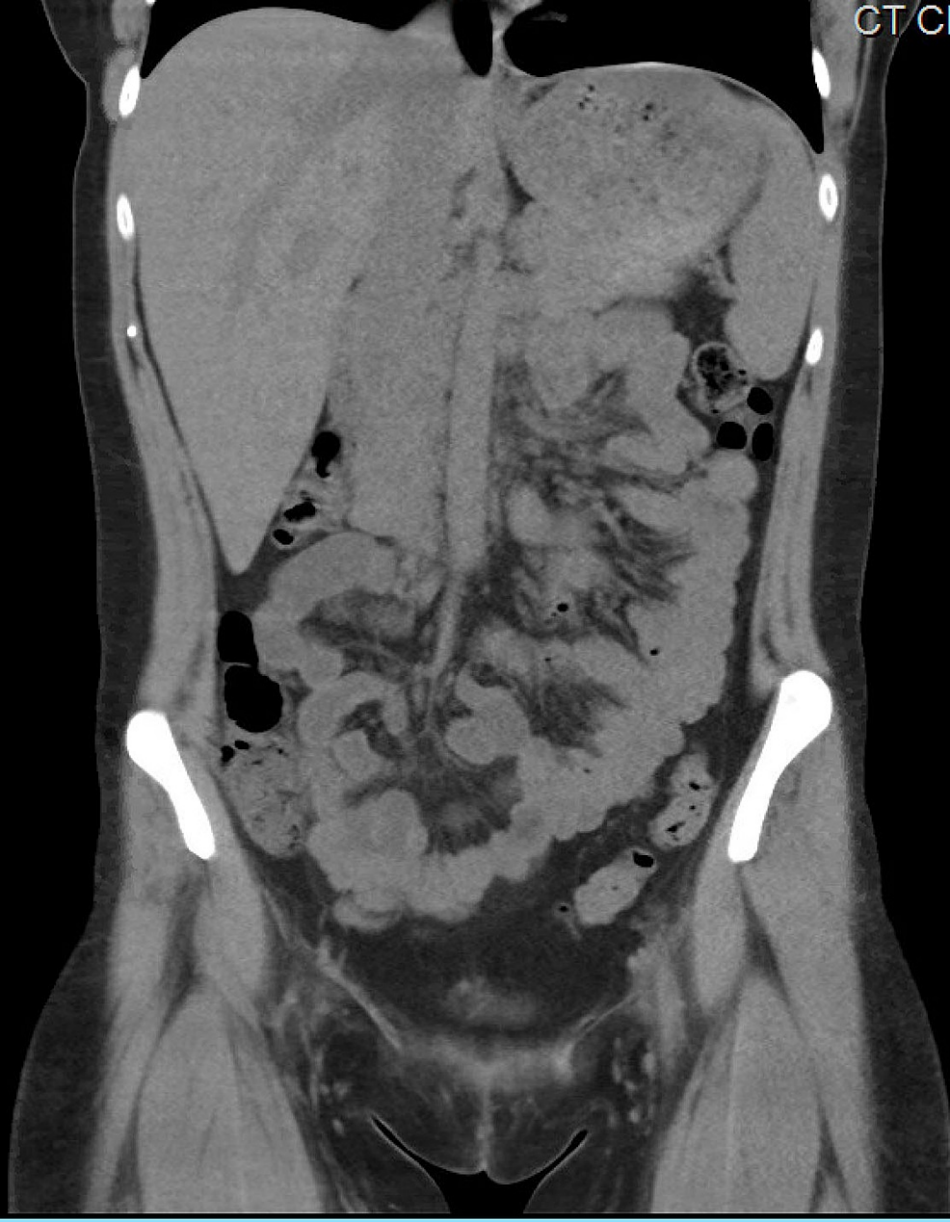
CT scan of the abdomen shows no masses to suggest abdominal malignancy
